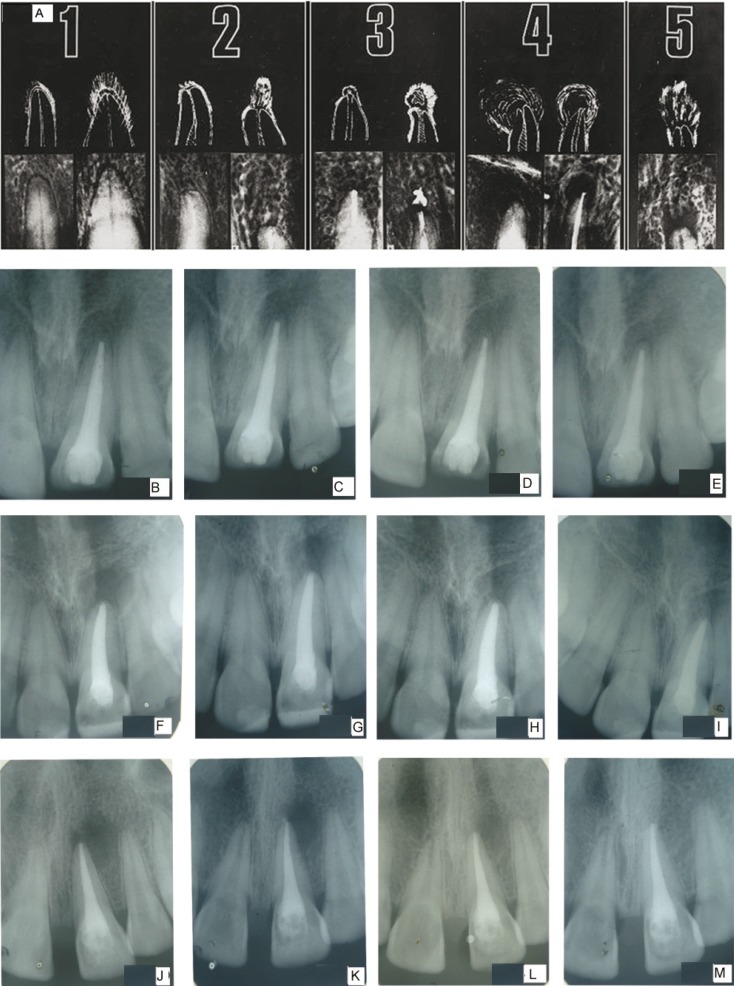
A. Periapical index assessment; b. Calcium hydroxide obturation; c. Calcium hydroxide 6 months; d. Calcium hydroxide 12 months; e. Calcium hydroxide 18 months; f.Triple antibiotic paste obturation; g. Triple antibiotic paste 6 months; h. Triple antibiotic paste 12 months; i. Triple antibiotic paste 18 months; j. Photoactivated disinfection obturation; k. Photoactivated disinfection 6 months; l. Photoactivated disinfection 12 months; m. Photoactivated disinfection 18 months.
